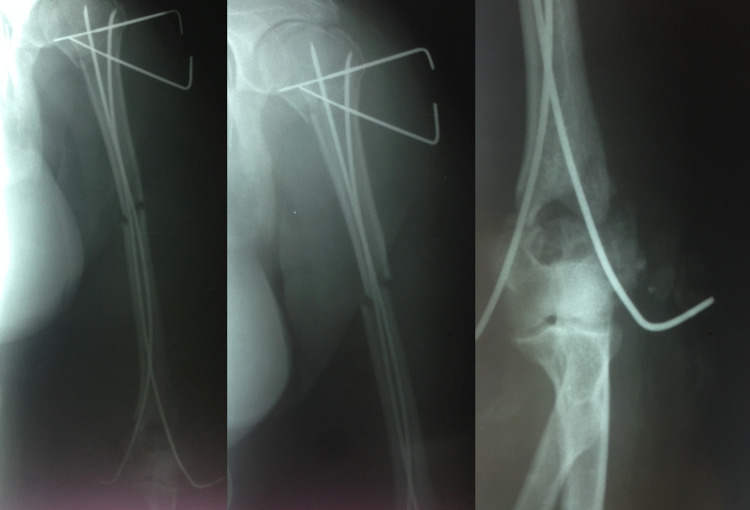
Immediately postoperative X-rays of the humerus - Kirschner wires were induced percutaneously with the outer edge outside of the body and it remained there for four weeks
